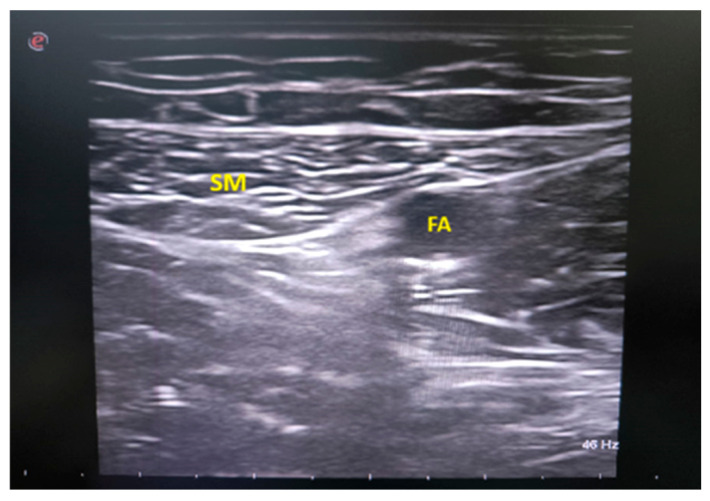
Ultrasound Image of the Distal Adductor Canal Block (FA: Femoral artery, SM: Sartorius muscle).
